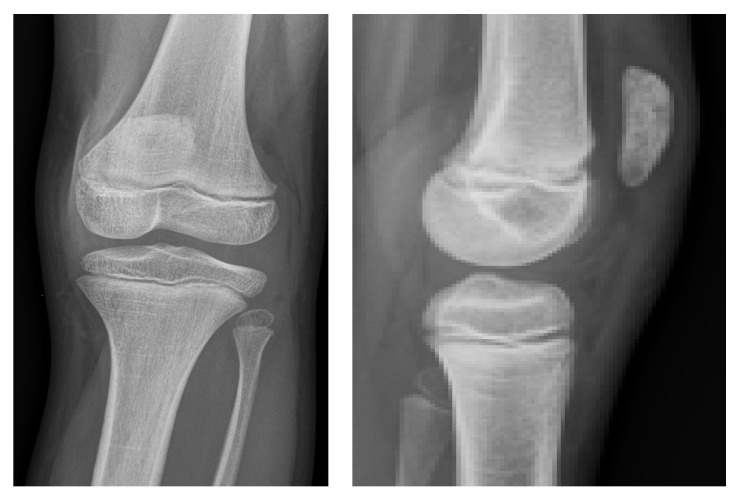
Radiographs of the knee joint 8 weeks after the first symptoms. A hypodense area is visible at the upper pole of the patella.
